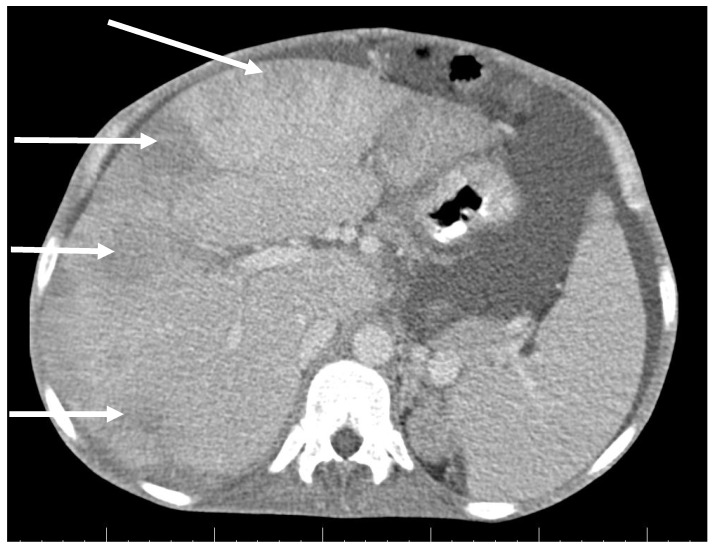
Abdominal CT scan shows multiple lesions in the liver (arrows).
